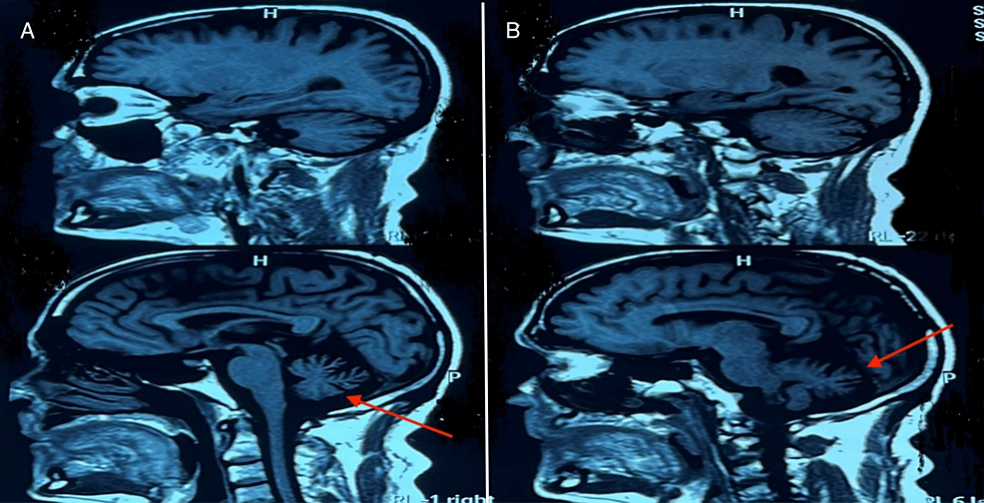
Brain MRI findings showed cerebellar atrophy with a vermian predominance (red arrows) in two patients (A and B)
